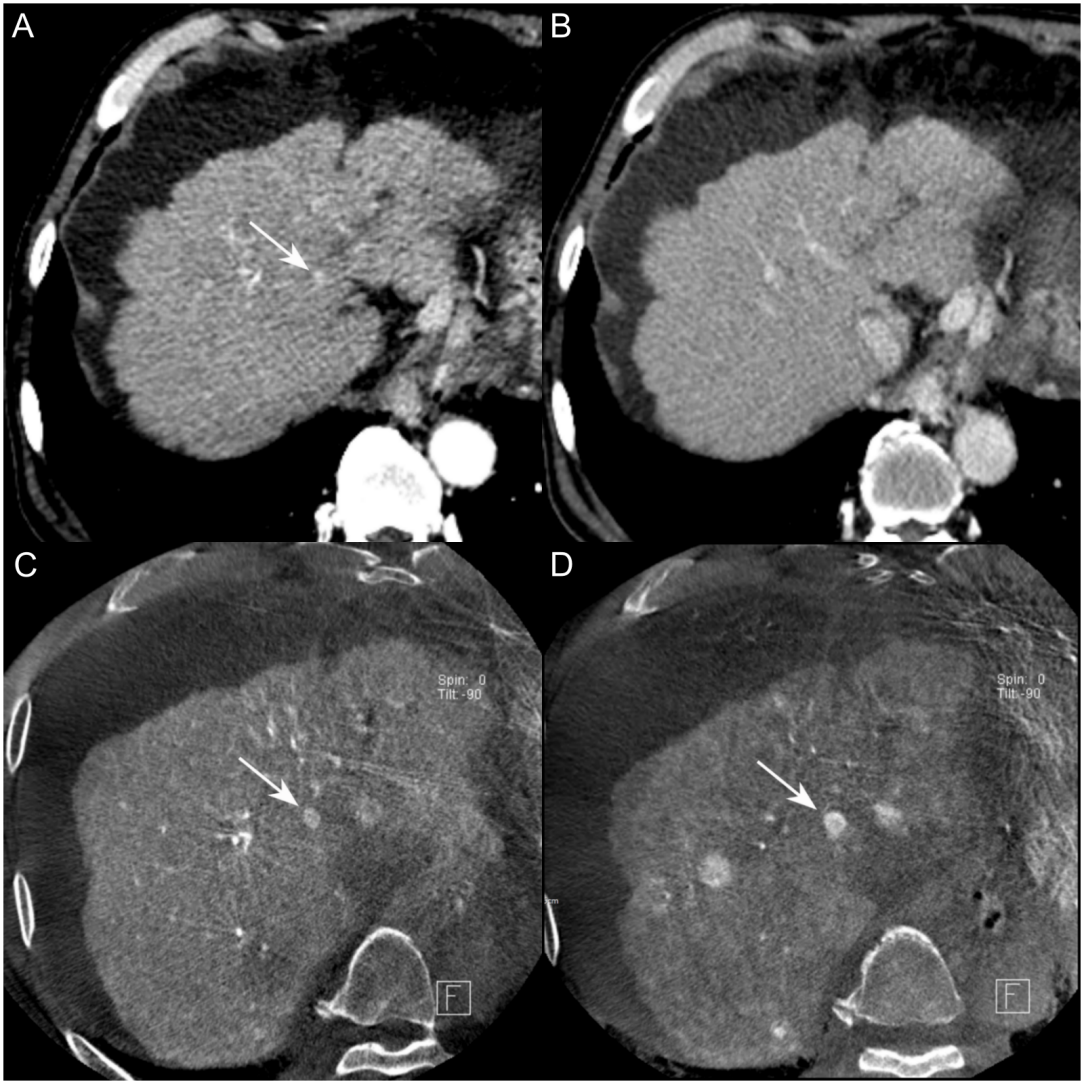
76-year-old man with type II HCC smaller than 1 cm in diameter. A-B, On the contrast-enhanced CT in arterial (A) and delayed (B) phases images, a 7 mm sized nodule in S1 of the liver showed arterial enhancement without washout (type II, arrow). This lesion was not mentioned on formal CT report, and was not detected by both reviewers, neither. C-D, CBCT-HA images on initial TACE session (C) and 6 months later (D). A small hypervascular nodule showed interval growth (11 mm, arrow) on follow up CBCT-HA. This nodule showed interval growth with typical enhancement pattern of HCC on the follow-up CT (not shown).
